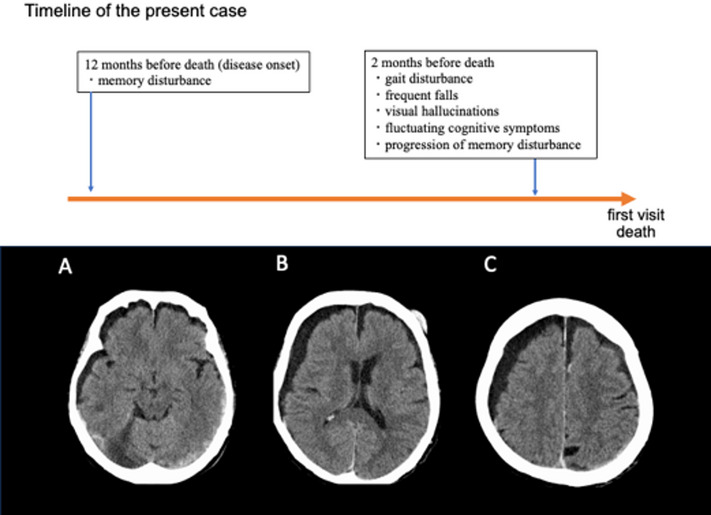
Timeline of the present case and brain computed tomography. The upper panel shows the timeline of the present case, and the lower panel shows brain CT images (A–C). A Midbrain atrophy was not notable. Traumatic subarachnoid hemorrhage was observed primarily in the left occipito-temporal lobe. B A subcutaneous hematoma was observed in the left frontal region. B, C A right chronic subdural hematoma was observed, with slight medial shift of the right cerebral hemisphere
